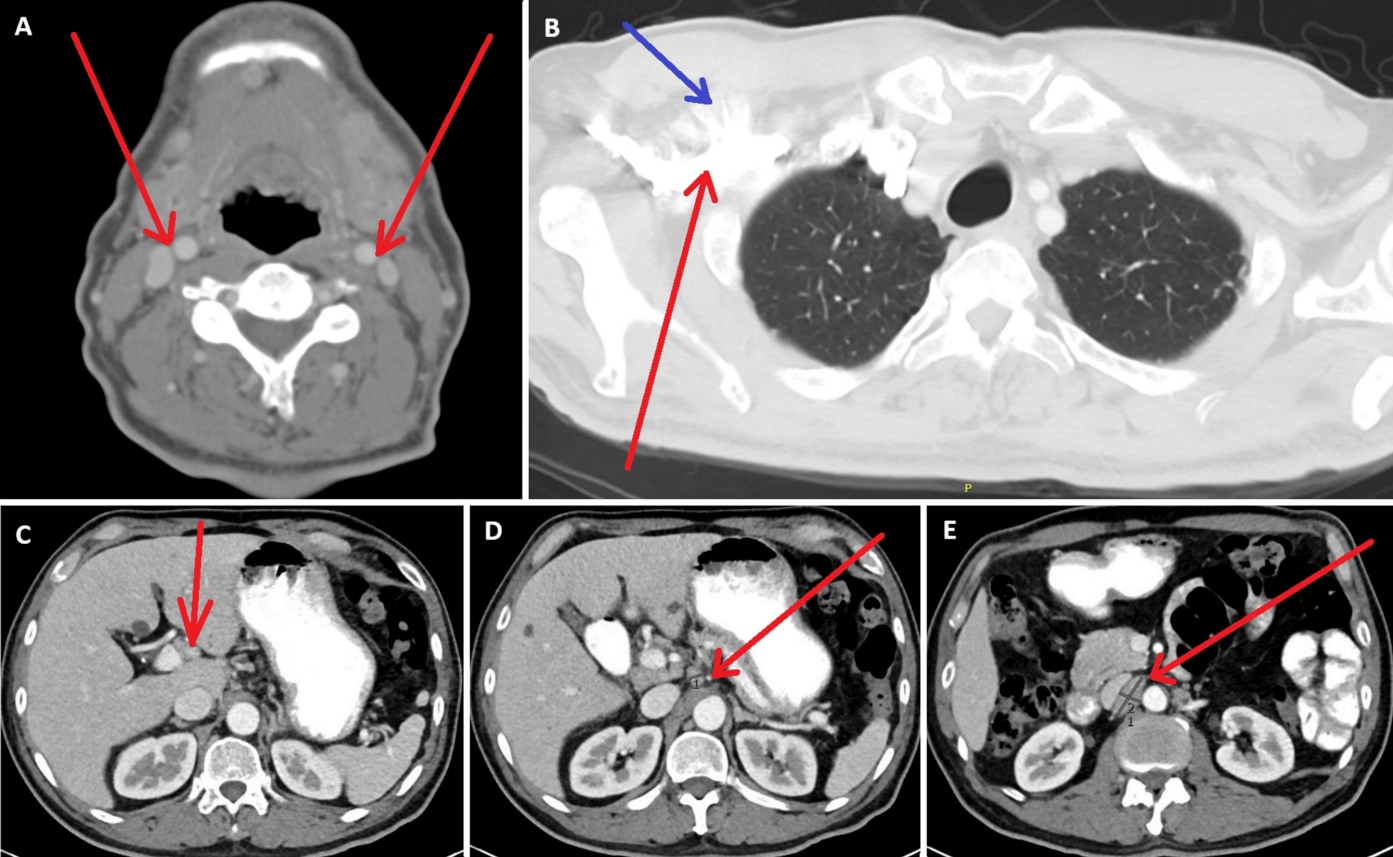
CT imaging of the neck, chest, and abdomen demonstrating multistation lymphadenopathy. (A) Neck CT demonstrating bilateral upper cervical chain adenopathy (red arrows). (B) Chest CT demonstrating several prominent and enlarged right axillary lymph nodes (red arrow) with adjacent fat stranding (blue arrow). (C-E) CT abdomen demonstrating multistation retroperitoneal and abdominal lymphadenopathy (red arrows), including an enlarged periportal lymph node (C), gastroduodenal and gastrohepatic lymph nodes (D), and bulky right para-aortic lymphadenopathy (E).
